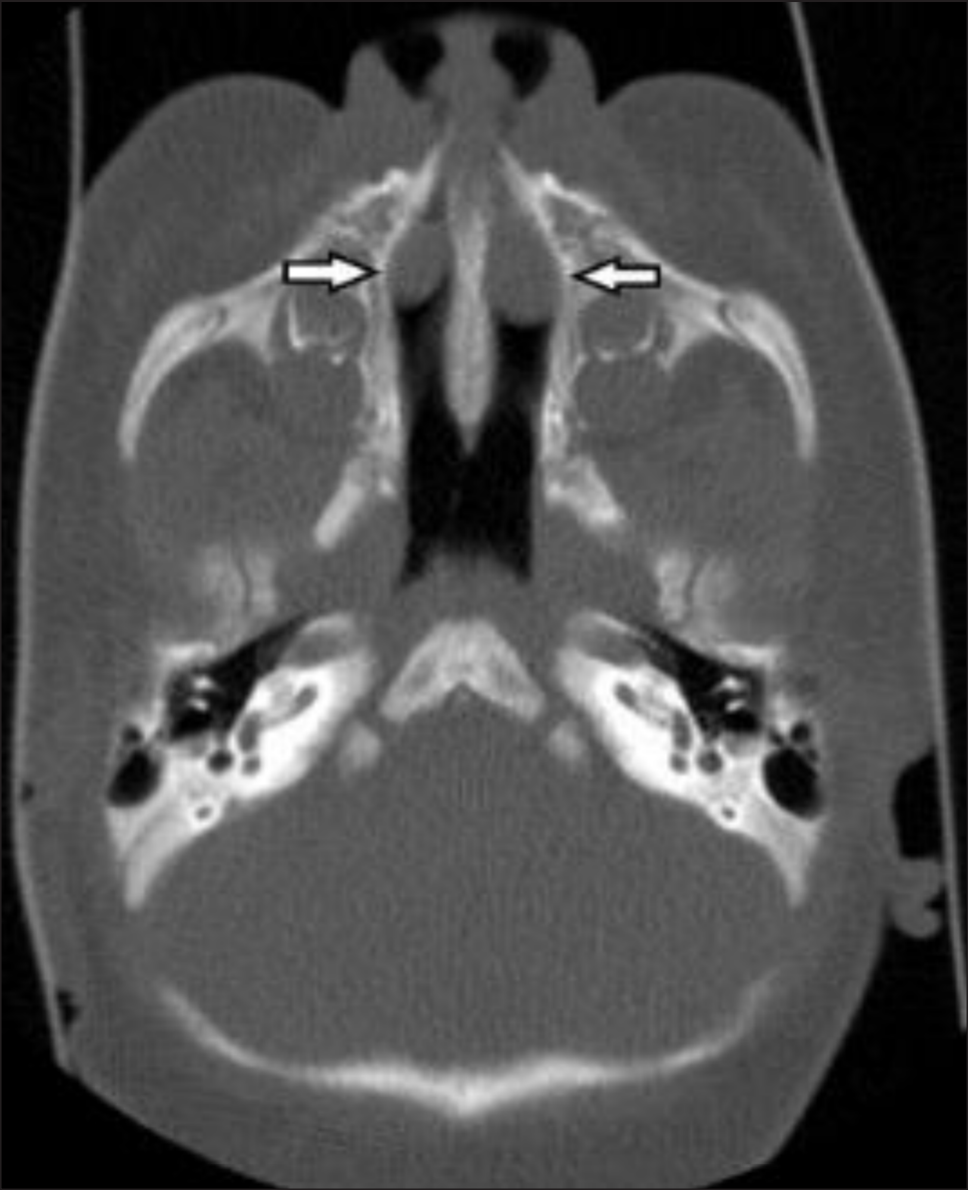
Axial CT image in bone window at the level of the nasal cavity shows the bilateral anterior intranasal mass, compatible with a bilateral dacryocystocele.
